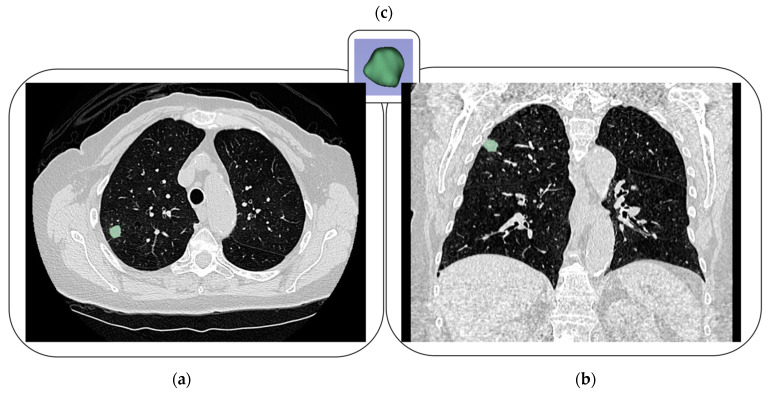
Example tumor segmentation (green) shown on (a) axial and (b) coronal CT views, with (c) a 3D volume rendering.
